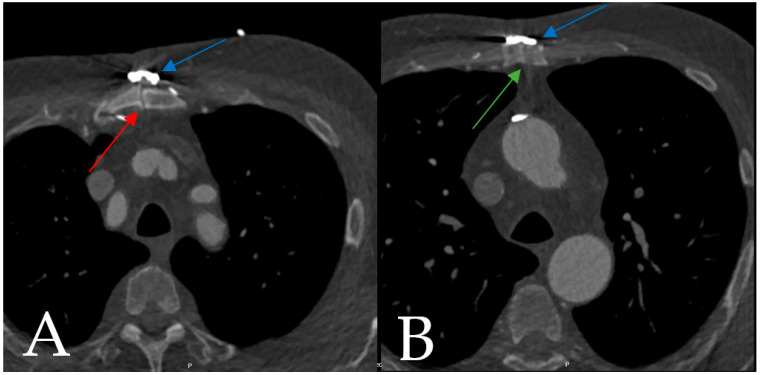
Postoperative computed tomography of a 76-year-old male 3 months after aortic valve replacement and replacement of the ascending aorta, as well as enhanced sternal closure using the SL360. (A) Red arrow: 3 mm mal-union zone in the region of the manubrium sterni. (B) Green arrow: consolidated bone in the region of the 3rd intercostal space. (A,B) Blue arrow: SL360 enhanced sternal closure system.
